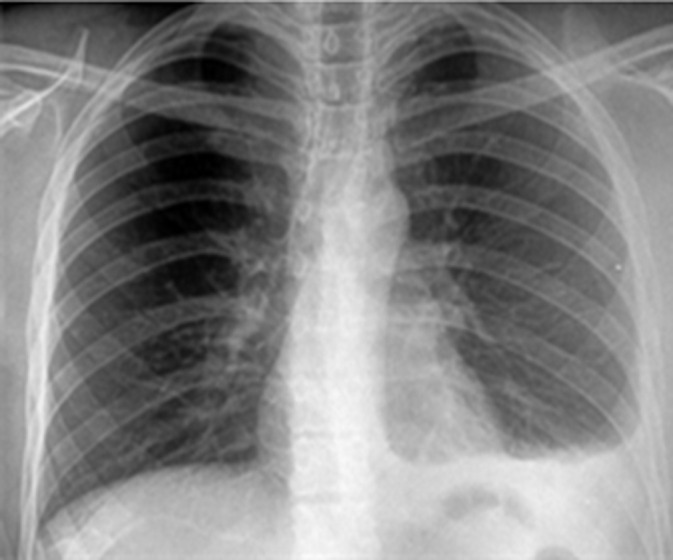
chest radiography showing minimal left-sided pleural effusion
